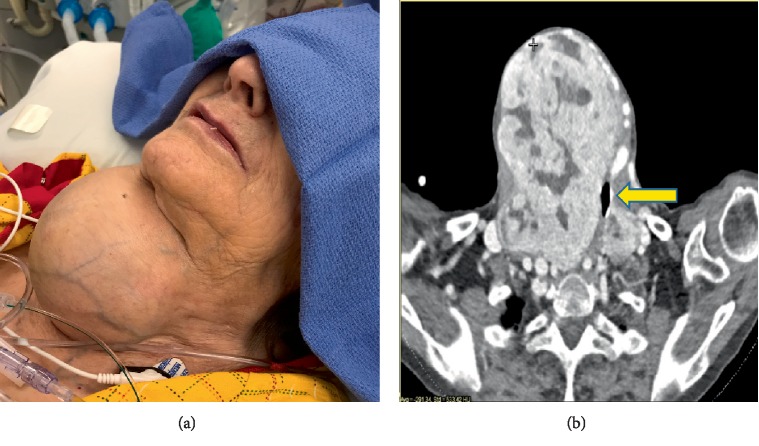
Image demonstrating the presence of the large clinically palpable goiter (a). CT scan showing trachea at its narrowest diameter (yellow arrow- (b)).
